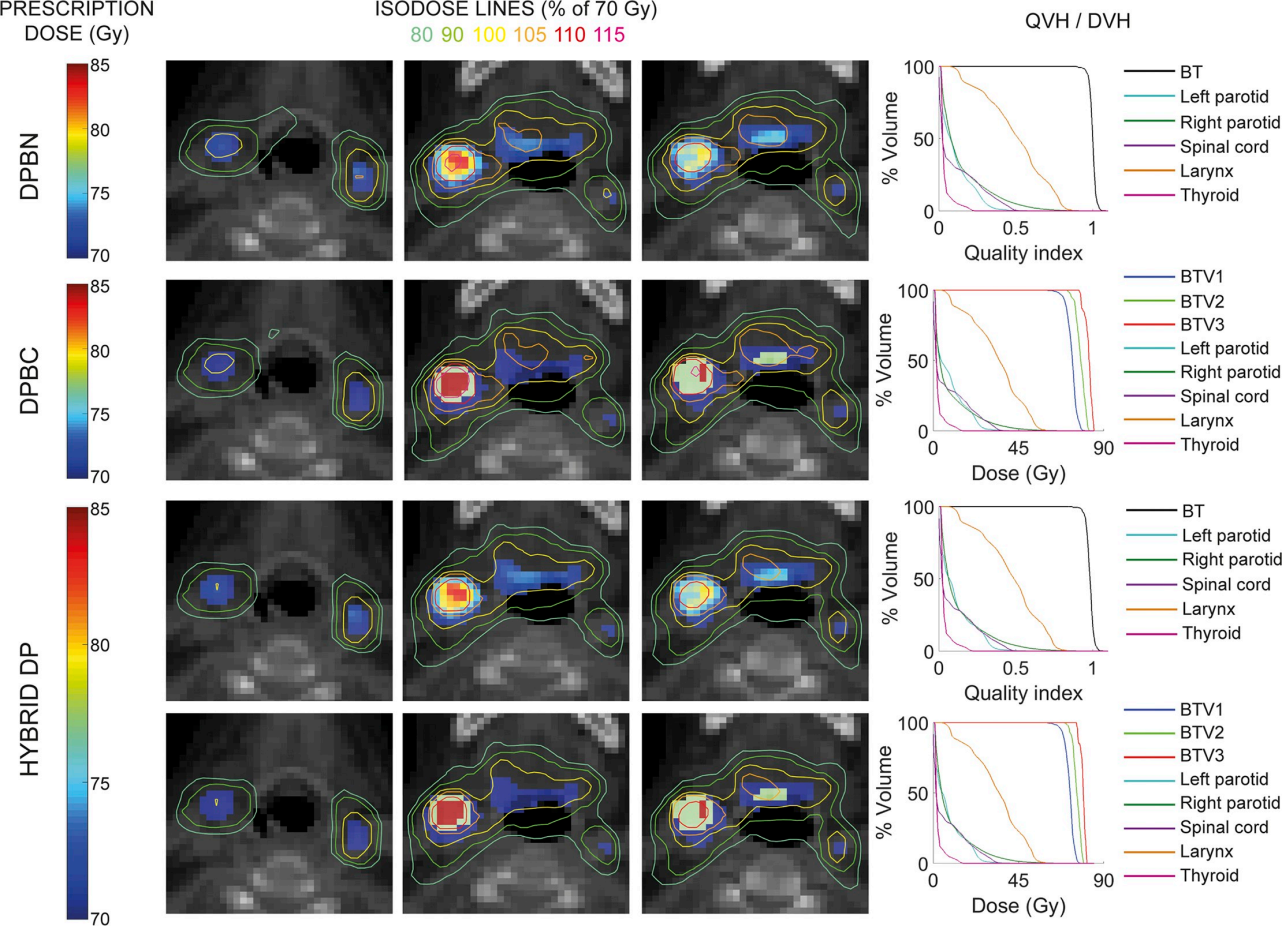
DPBN and DPBC plans to solve dose prescriptions based on numbers and on volumes, respectively, and HYBRID DP planning to solve both dose prescriptions. The corresponding isodose lines show the relative dose distributions as a percentage of the minimum prescription value (70 Gy) for the three plans. The isolines are visualized over both prescription approaches on CT image. DVHs and QVHs are presented at the left for each planning solving the corresponding dose prescription.
